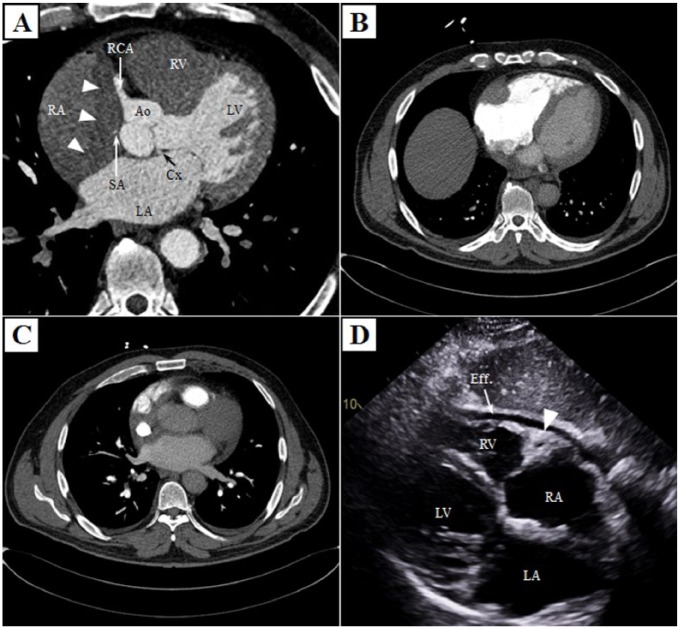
Chest computed tomography and transthoracic echocardiography. Cardiac computed tomography (with contrast timing chosen to best delineate the coronary arteries) demonstrated infiltrative soft tissue involving the right atrioventricular groove and upper portion of the interatrial septum encasing the right coronary artery and the sinoatrial node branch (A and supplementary video 1 [supplementary video is available in the online version of the article]). Chest computed tomography using the pulmonary embolism protocol allowed better visualization of the infiltrative soft tissue mass involving the right atrioventricular groove and superior aspect of the atrial septum surrounding the superior vena cava at its junction with the right atrium (B and C). Subcostal costal view of transthoracic echocardiography demonstrated a small pericardial effusion along with the soft tissue mass in the right atrioventricular groove encasing the right coronary artery (D) (Ao, aortic root; Cx, circumflex coronary artery; Eff, effusion; LA, left atrium; LV, left ventricle; RA, right atrium; RCA, right coronary artery; RV, right ventricle; SA, sinoatrial node branch).
